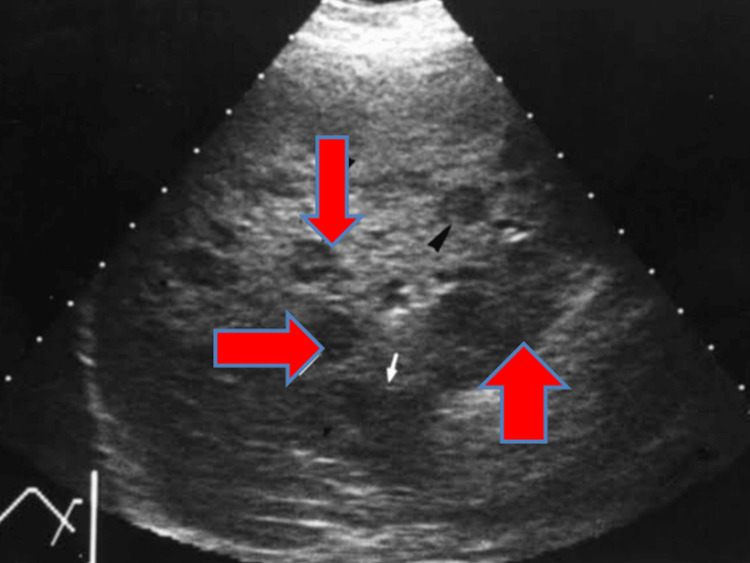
Ultrasound of the abdomen showing an enlarged spleen with clustered cystic spaces without internal vascularity.
